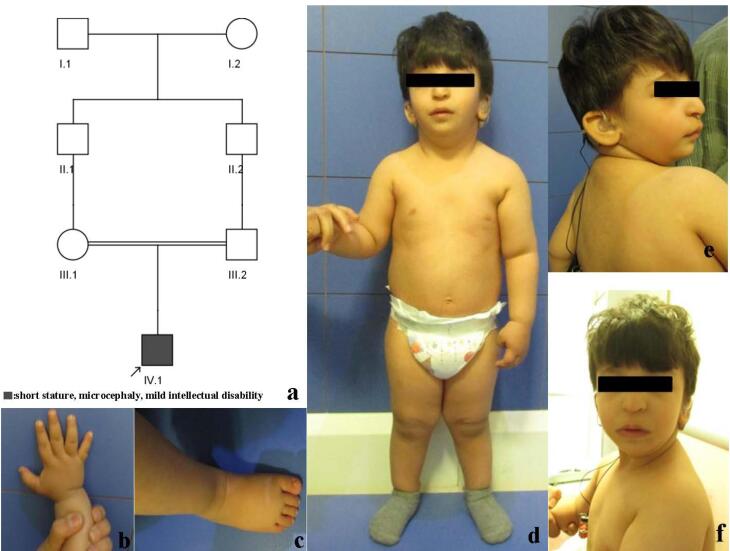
Paraclinical examination: Brain CT was performed at 2.5 months but was not very helpful since fontanels were closed. Ultrasound examination of the pelvis, kidneys, bladder, testes and inguinal canal was performed at 1 month and 3 days. The hip was normal and did not show dysplasia. The kidneys were normal in size and shape.
